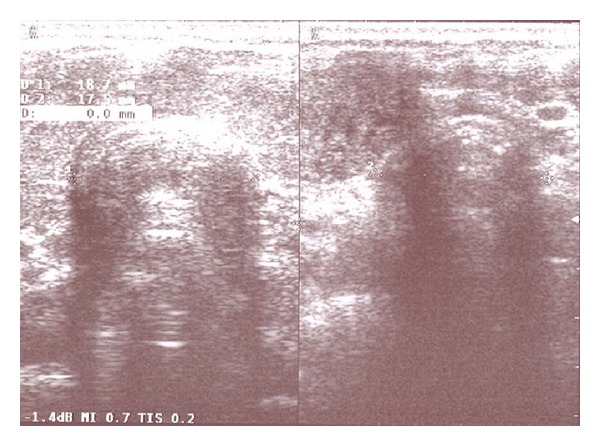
Neck and thyroid ultrasound examination (Case  2): trachea with a reduction of the transverse diameter (17.5 mm) in the distal cervical tract and dislocation to the left of the trachea.
